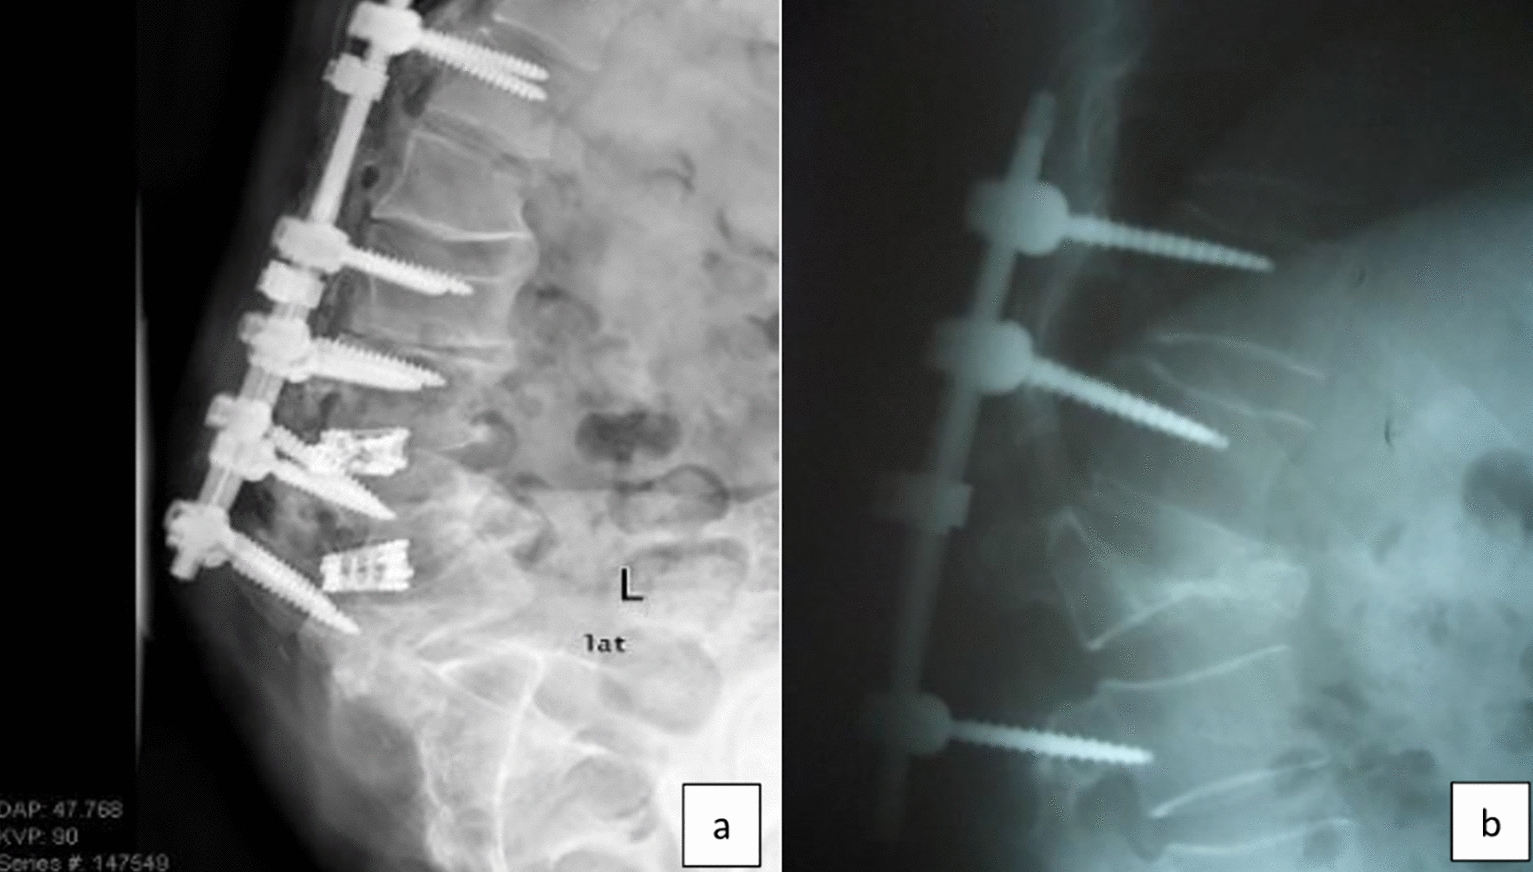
a, b Lateral X-ray shows screw pull out
